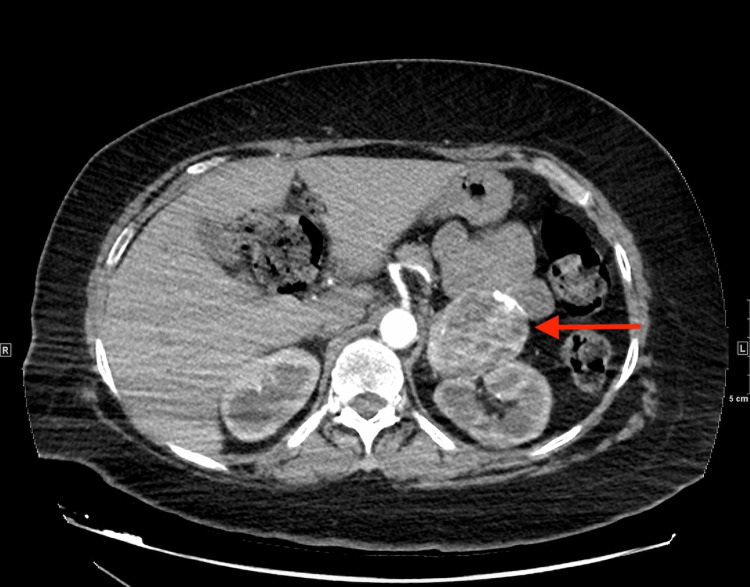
Axial view, CTA: showing a well circumscribed, left adrenal mass (red arrow) CTA: computed tomography angiography
